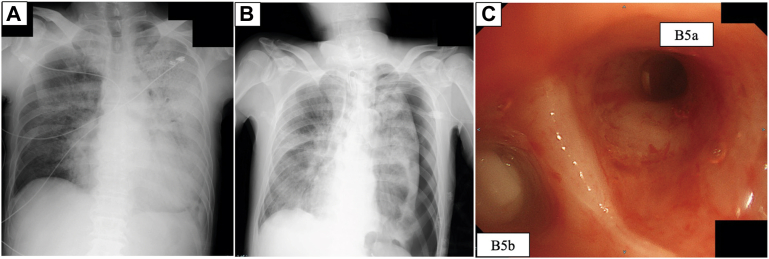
(A) Chest radiograph on hospital day 6 showing extensive infiltration and reduced aeration in the left lung field. (B) Chest radiograph on hospital day 19 showing a left-sided pneumothorax. (C) Bronchoscopic view on hospital day 20 showing endobronchial Watanabe spigots placed in B5a and B5b.
